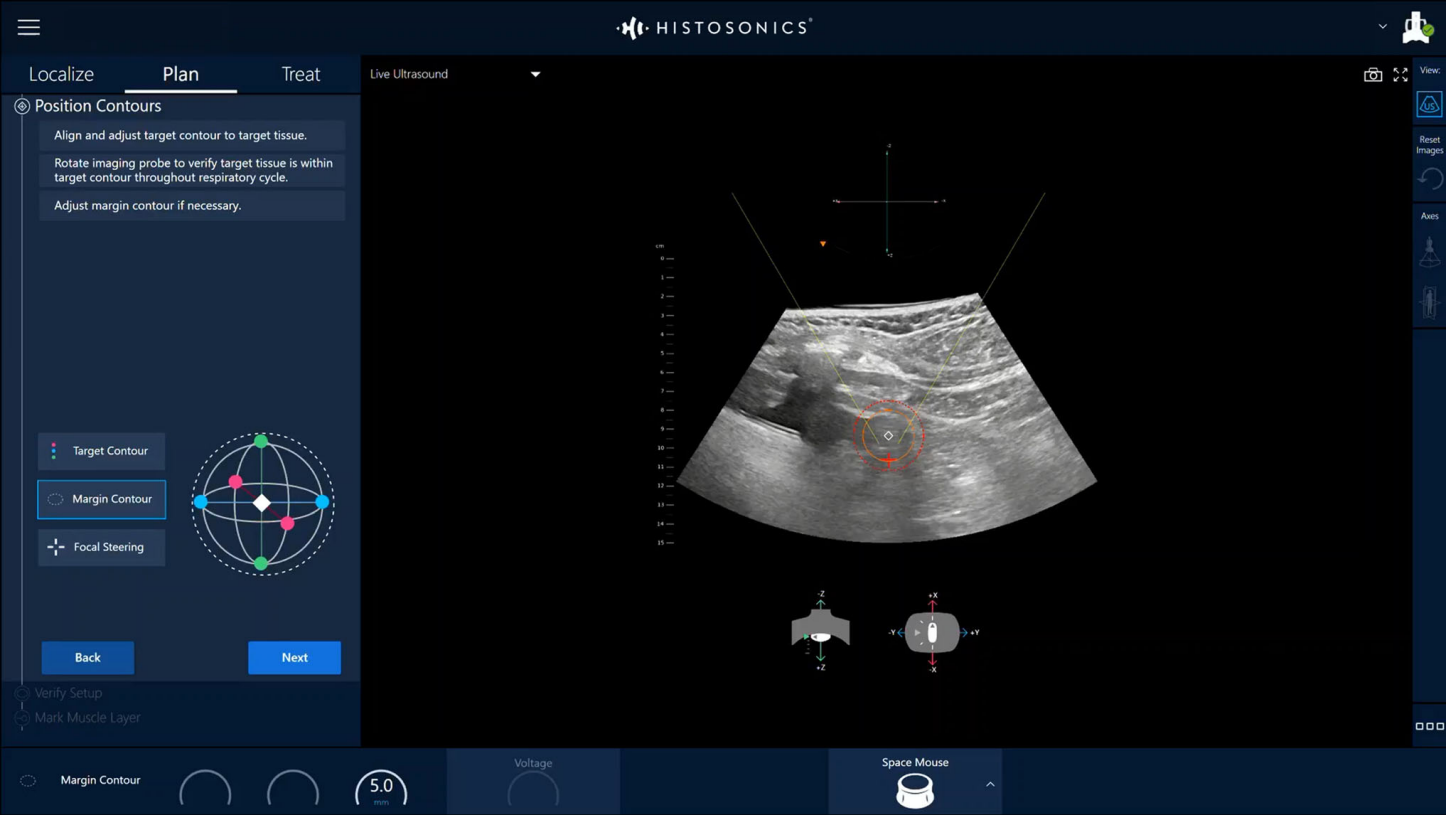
HistoSonics System User Interface. The HistoSonics System user interface provides the physician access to reference images and enables targeting, planning and treatment in real time. The workflow panel (displayed on the left) guides the physician during the localize, plan, and treat phases. The live ultrasound is displayed in the middle; using the workflow, the physician defines the PTV (target tumour [orange ellipse] and margin [red ellipse])
